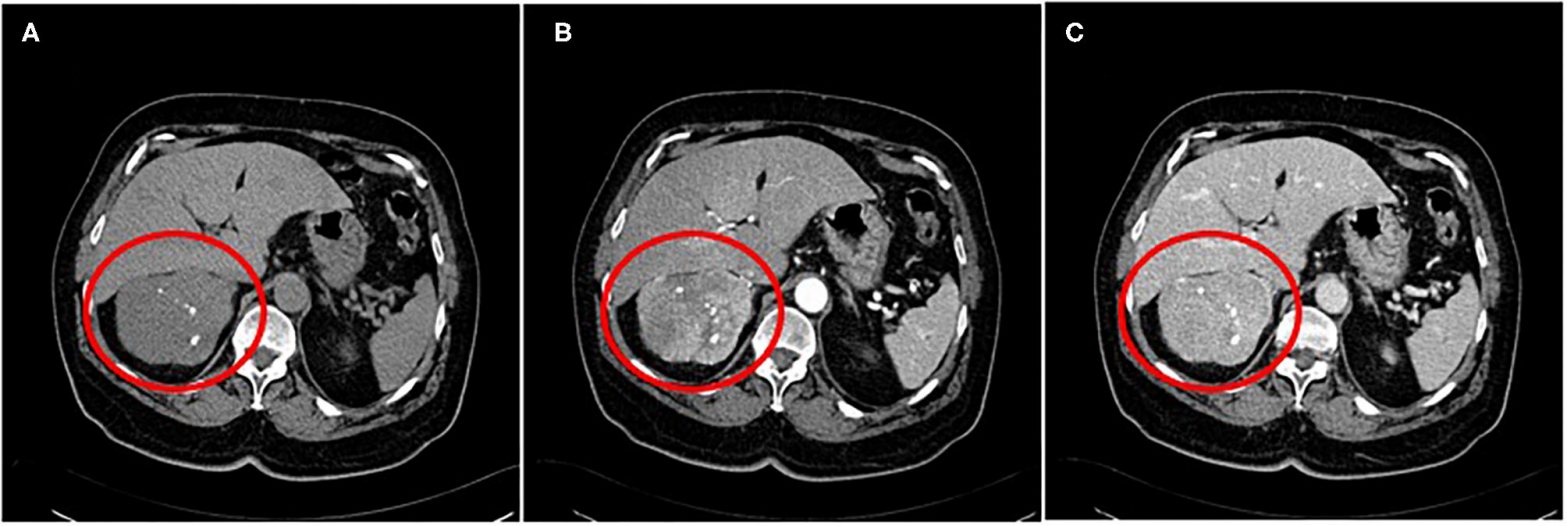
A contrast-enhanced abdominal CT scan showing a quite large heterogenous mass with focal calcifications (A) ~9 cm in size. The lesion had not only arterial (B) but also venous enhancement, while some areas remained nonenhanced, possibly necrotic (C).
